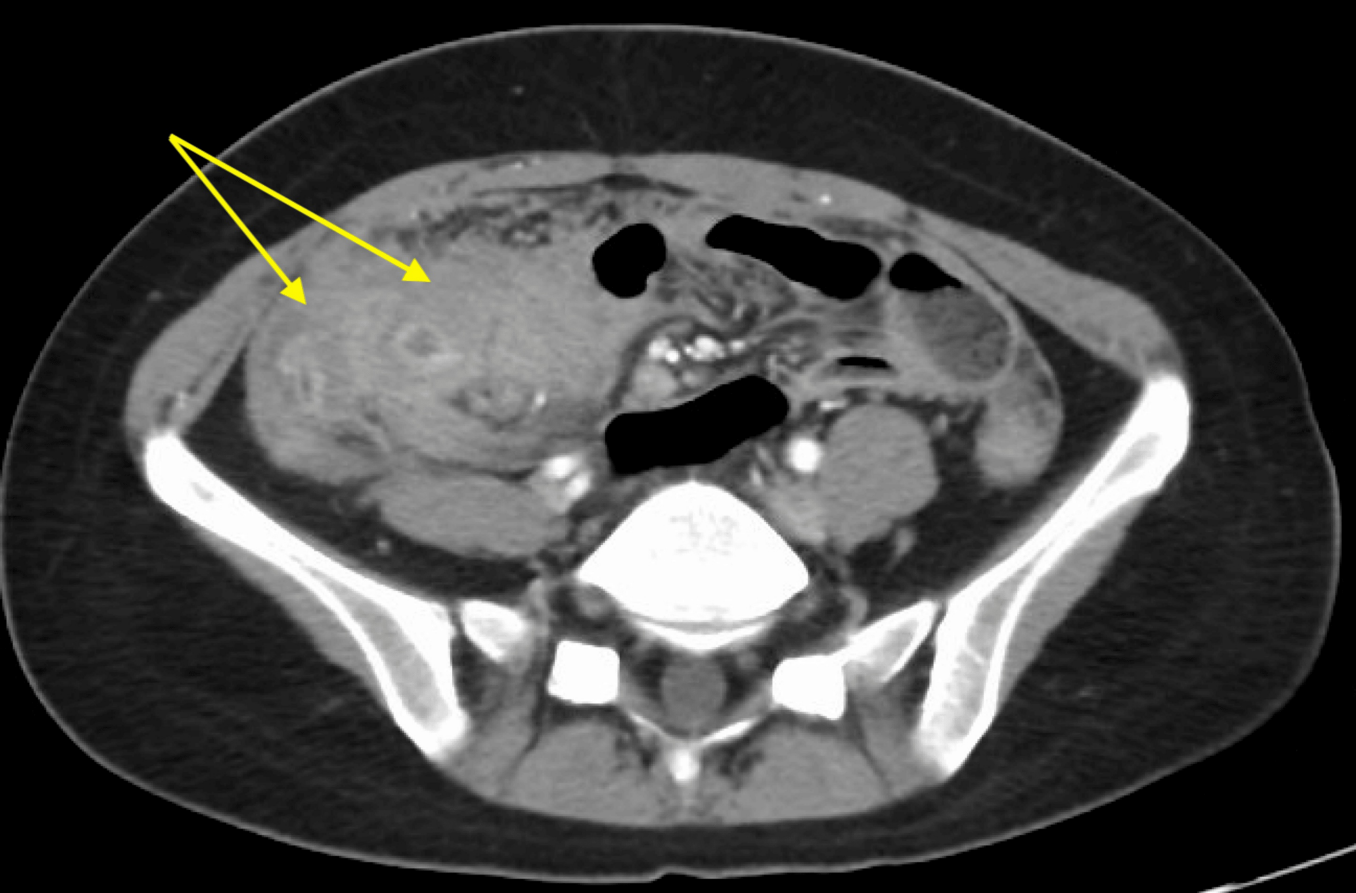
Axial view of CT abdomen and pelvis demonstrating inflammatory change
